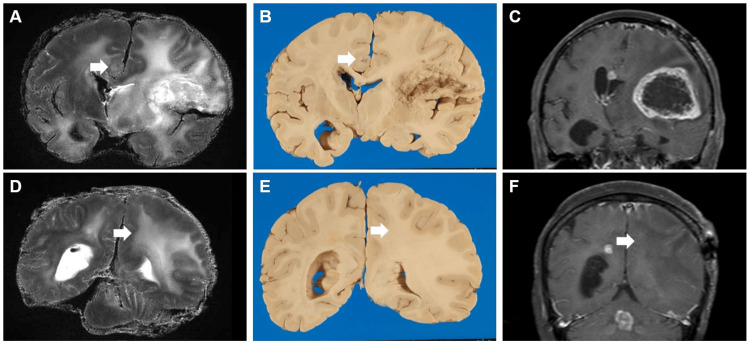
Comparison of premortem and postmortem MRI findings (Case 3) A, B, and C are coronal MRI images of the same cutting plane. A nodular lesion in the right cingulate gyrus with periarcuate in A and B (arrows). A: Postmortem T2-weighted images; B: Brain autopsy; C: T1 contrast image of the final premortem MRI scan. D, E, and F show coronal MRI images of the same cutting plane. T2 high-signal area extension is seen throughout the left hemisphere of the brain (arrows). D: Postmortem T2-weighted images; E: Autopsy of the brain; F: T1 contrast image of the final premortem MRI.
